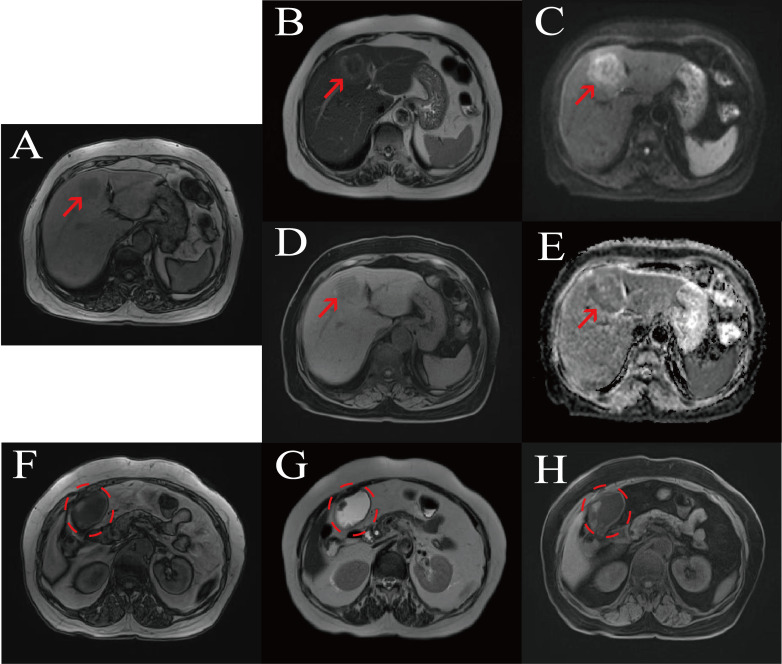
Non-contrast MRI. (A) T1w GRE (out-phase) sequence of the liver; (B) T2w sequence of the liver; (C) Diffusion-weighted imaging of the liver (b = 800 s/mm²); (D) T1w fat-saturated sequence of the liver; (E) ADC map of the liver; (F) T1w GRE (out-phase) sequence of the gallbladder; (G) T2w sequence of the gallbladder; (H) T1w fat-saturated sequence of the gallbladder.
